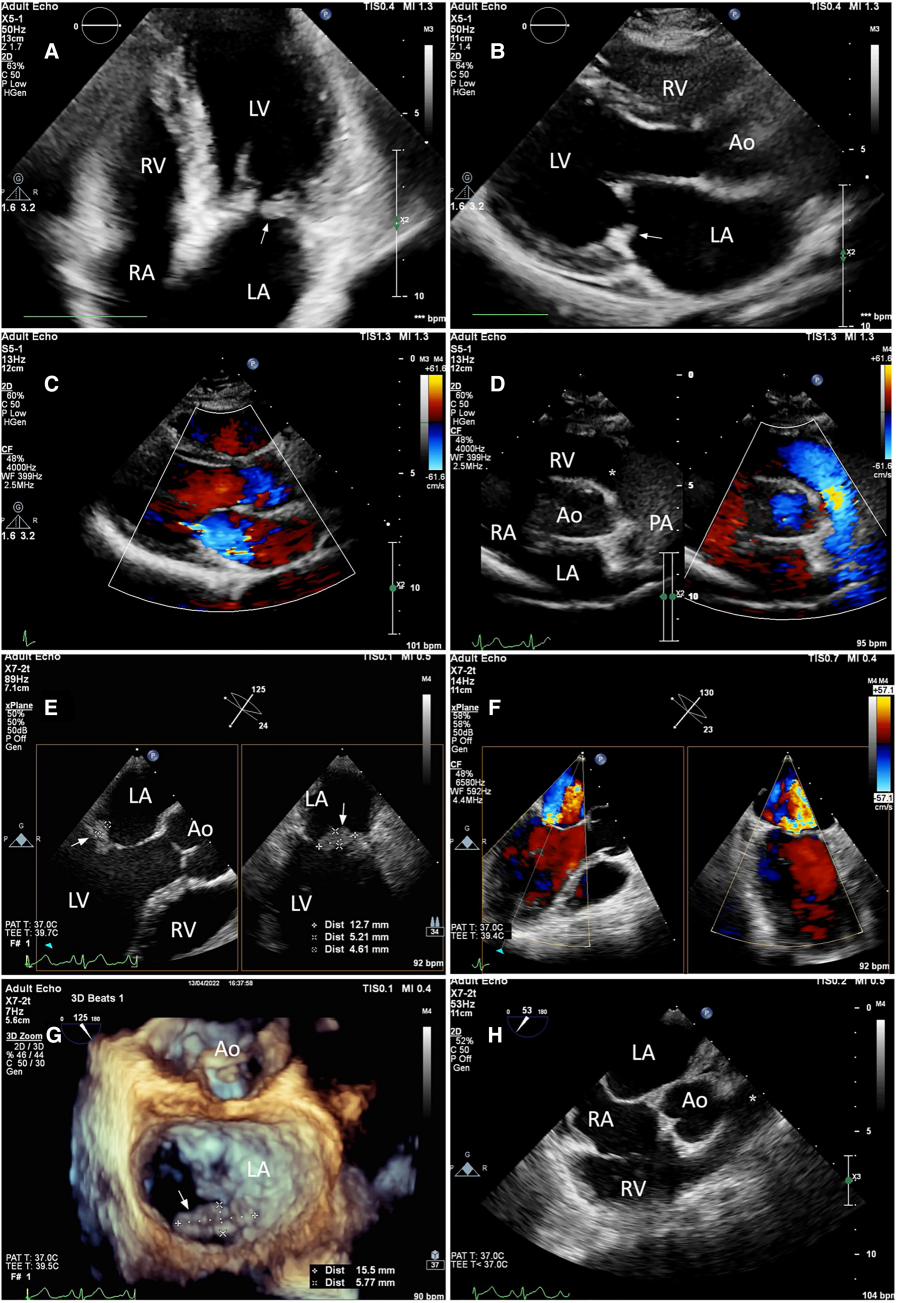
Still-frame images of the mitral and pulmonary valves on TTE (A–D) and TEE (E–H). (A,B) TTE apical four-chamber and parasternal long axis views revealed a well-circumscribed, sessile mass with heterogeneous echotexture which was firmly attached to the atrial side of the posterior leaflet of the mitral valve (white arrow). The mitral valve leaflets were thickened. (E) The maximum diameter of the mass was measured using 2D Xplane imaging at the mid-esophageal level. (G) From the left atrial perspective (en-face view), the vegetation was seen attached to the atrial side of the P2 and P3 portions of the posterior leaflet (white arrow) with 3-dimensional zoom-mode. (C,F) mild-to-moderate central mitral regurgitation was seen on both TTE and TEE with color Doppler. (D,H) The pulmonary valve as visualized on the parasternal short-axis and mid-esophageal right ventricle inflow-outflow view was unremarkable (asterisk). Annotations: LA, left atrium; LV, left ventricle; RA, right atrium; RV, right ventricle; Ao, Aorta; PA, pulmonary artery.
